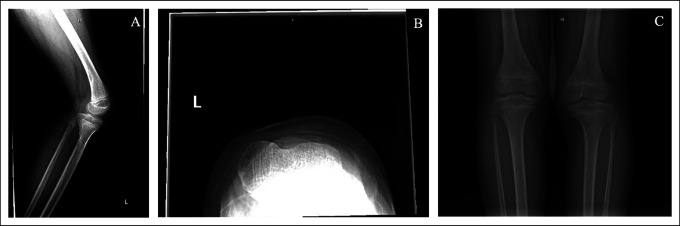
Radiograph images demonstrating bilateral absent patella. A, Left knee lateral view, (B) left knee sunrise view, and (C) bilateral weight-bearing AP view.
